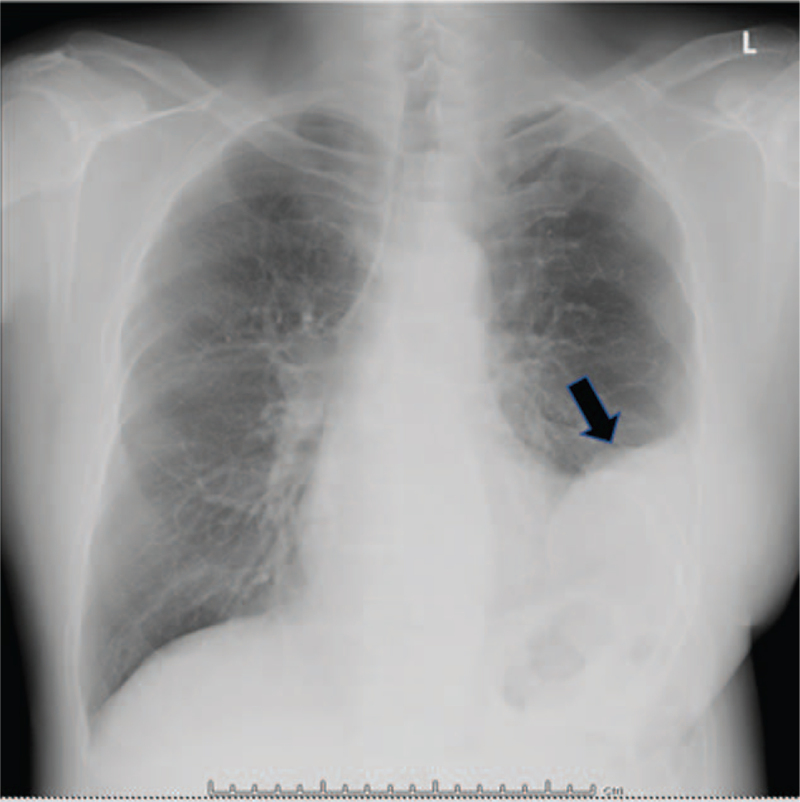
Infiltrative shadow in the left lower lung field upon chest radiography. The black arrowhead indicates that abdominal organs have prolapsed into the thoracic cavity.
